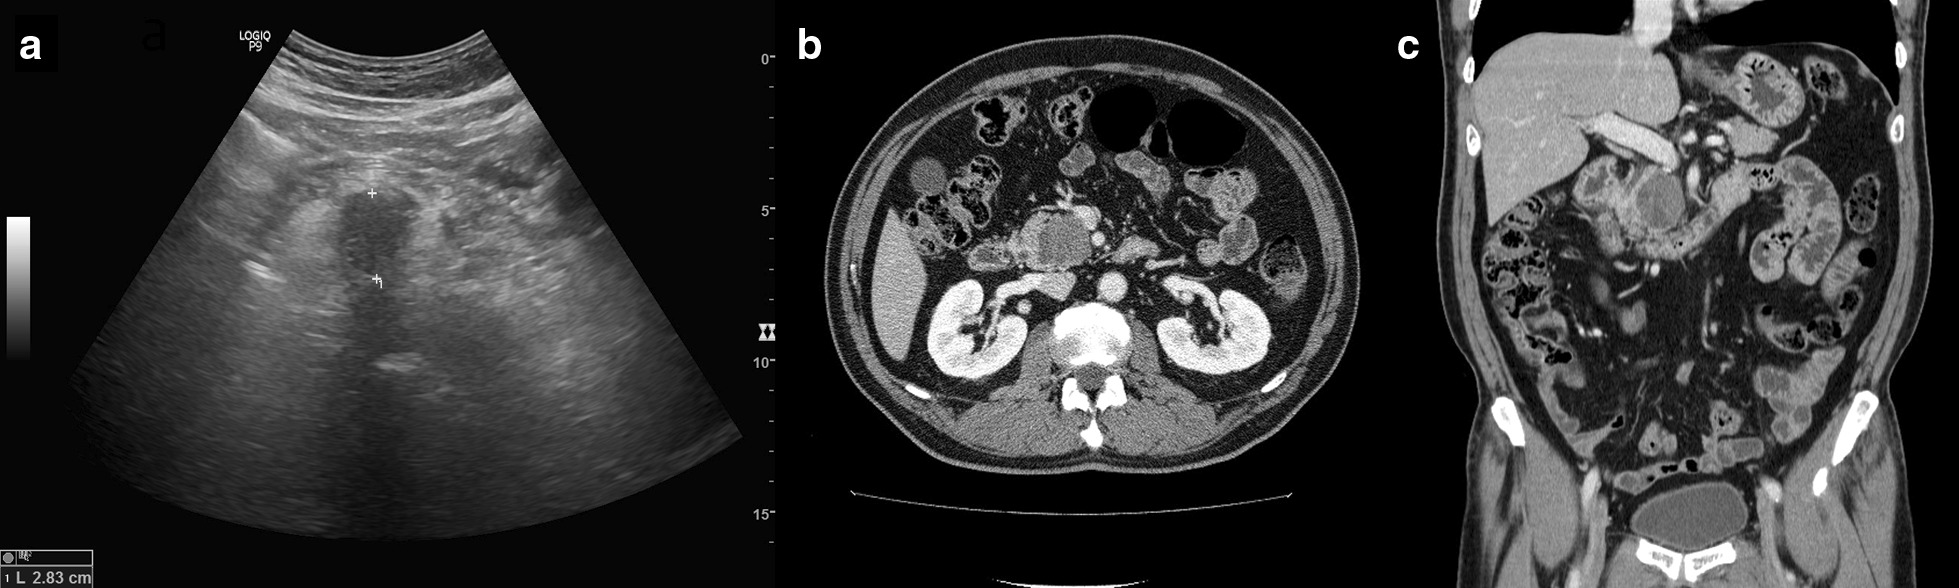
a Ultrasonography demonstrates a 2.8 cm sized hypoechoic mass lesion in the pancreatic head. b, c Computed tomography scan showing a 3.6 × 3 × 3.3 cm sized hypodense mass lesion in the pancreatic head region
